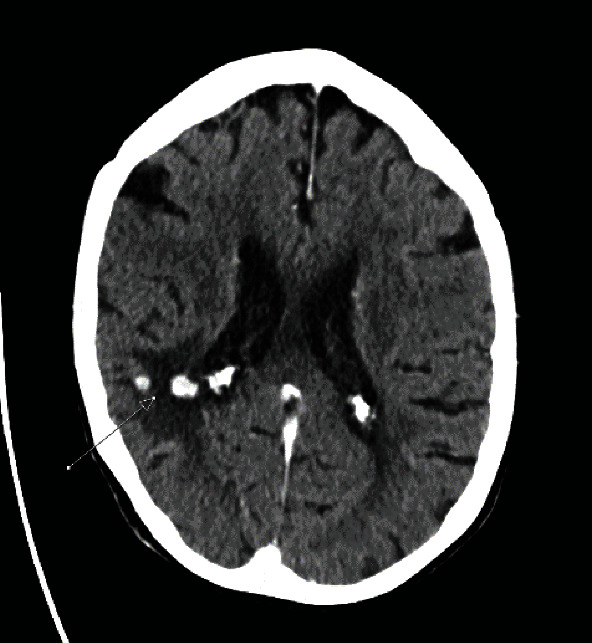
CT of the brain with contrast showing brain metastasis with surrounding edema.
